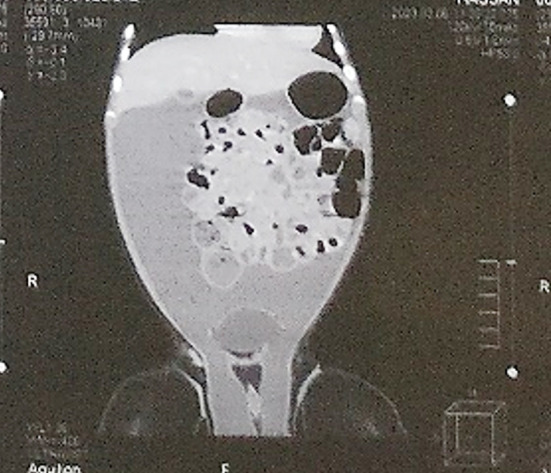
CT film showing extent of intraabdominal cystic lymphangioma into both scrotal regions.
